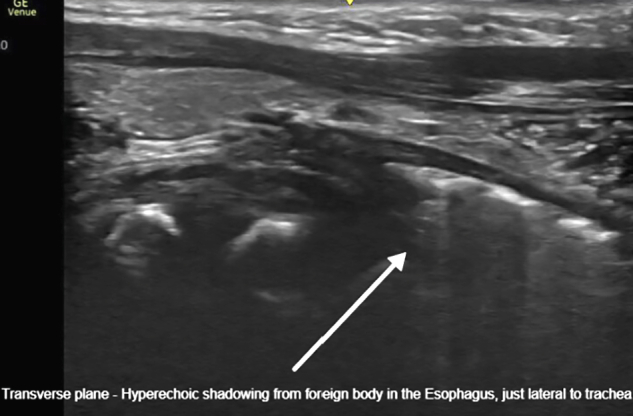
Longitudinal neck ultrasound confirming the foreign body within the upper esophagus (arrow).
